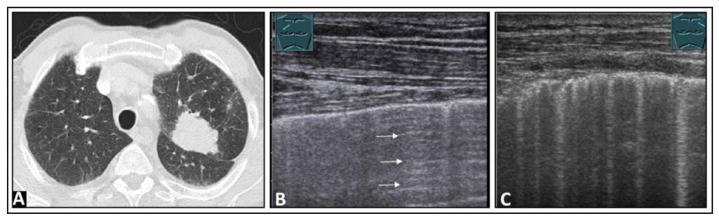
A patient with dyspnea on speaking, thoracic pain, and a confirmed left-sided intraparenchymal bronchial carcinoma located in the upper lobe, as seen on chest computed tomography (A). (B) On lung ultrasound, the right side displays an almost normal lung surface with A-lines (arrows). (C) Ventral to the centrally located lung tumor, given knowledge of the computed tomography findings, multiple vertical artifacts are evident in the form of B-lines and comet-tail artifacts, as seen in a focal interstitial pattern.
